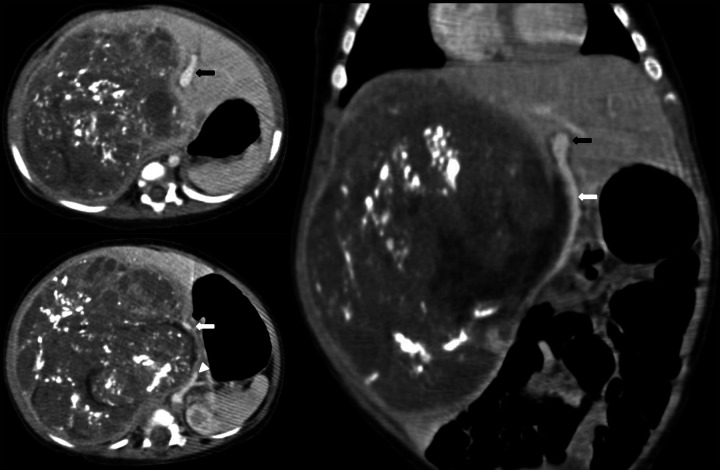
Post chemotherapy contrast-enhanced triphasic computed tomography, showing heterogeneously enhancing lesion with chunky calcification and necrotic non-enhancing spaces involving the entire right lobe with a large inferior exophytic component. The portal structures, aorta, and adjacent organs are grossly displaced by the mass. The main (white arrows), left branch of portal vein (black arrows), and common hepatic artery (arrow head) are stretched anteriorly along their medial edges while the right branch and inferior vena cava are not visible due to compression by the lesion
